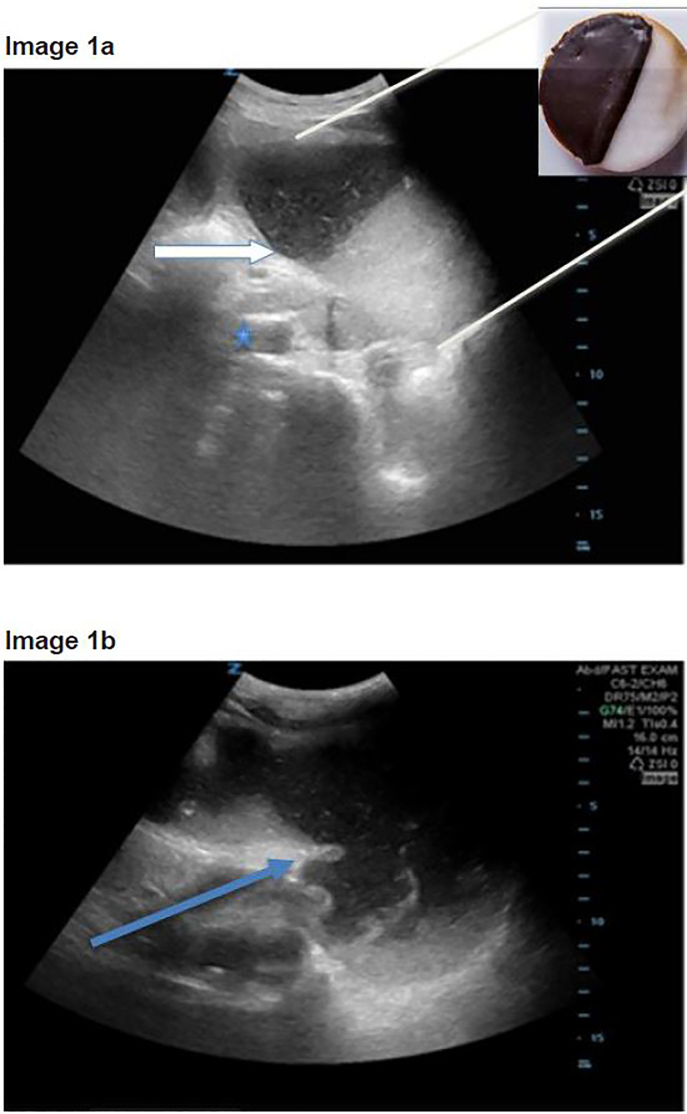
a) Sagittal orientation point-of-care ultrasound (POCUS) with “black-and-white cookie” sign seen by white arrow with division of stomach contents. Inferior vena cava (blue star) seen distal to the stomach; b) POCUS of dilated, fluid-filled stomach with thickened stomach wall (blue arrow).
